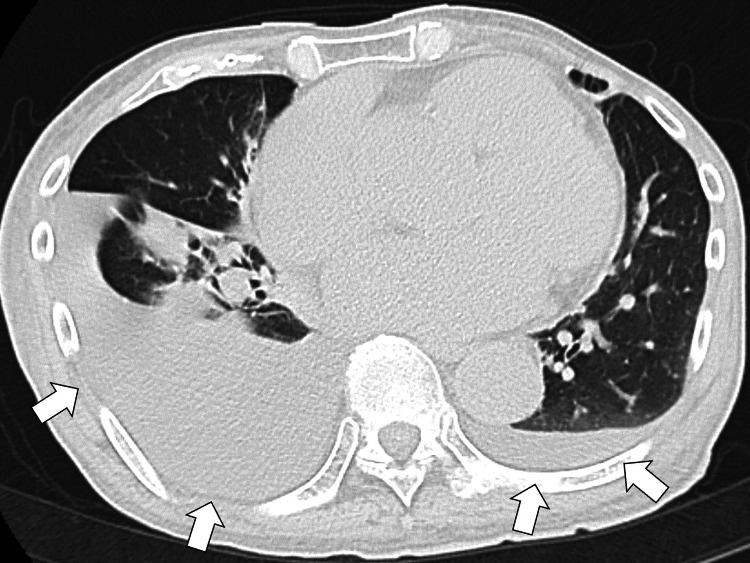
Thoracoabdominal computed tomography shows bilateral pleural effusions with no pleural or pericardial thickening (white arrows).
